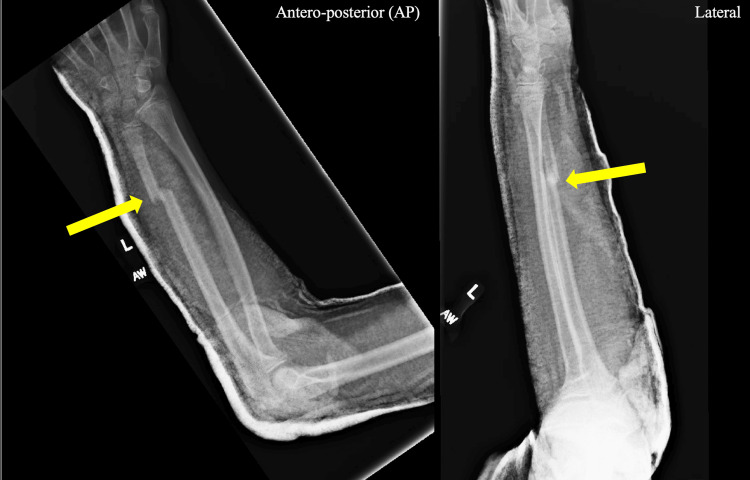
Anteroposterior (AP) and lateral X-ray imaging The arrows point to a displaced distal ulna fracture with an overlying splint
